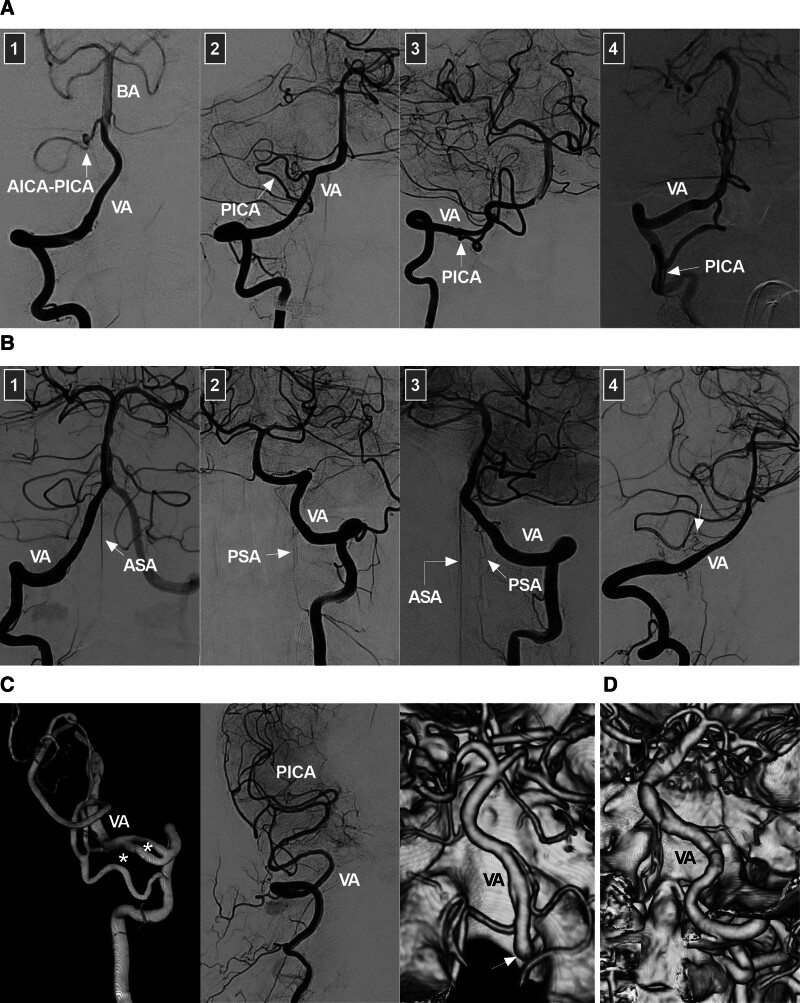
Intracranial VA anatomy and anomalies. (A) PICA anatomy: panel 1: DSA image showing the PICA and AICA sharing a common trunk (arrow); panel 2: DSA image showing the PICA (arrow) emerging from the distal VA; panel 3: DSA image showing the PICA (arrow) emerging from the intracranial proximal VA; panel 4: DSA image showing the PICA (arrow) emerging from the extracranial VA. (B) ASA and PSA anatomy: panel 1: DSA image showing the ASA (arrow) emerging from the VA; panel 2: DSA image showing the PSA (arrow) emerging from the VA; panel 3: DSA image showing the ASA and PSA (arrows) emerging from the VA; panel 4: DSA image showing a perforating artery (arrow) emerging from the VA. (C) VA anomaly: left panel: DSA image showing 2 fenestrations (asterisks) of the VA; middle panel: DSA image showing a VA terminating at the PICA; right panel: CTA image showing a VA emerging from the primitive hypoglossal artery (arrow). (D) CTA showing VA dolichoectasia, a form of vertebrobasilar dolichoectasia. AICA = anterior inferior cerebellar artery, ASA = anterior spinal artery, BA = basilar artery, CTA = computed tomography angiography, DSA = digital subtraction angiography, PICA = posterior inferior cerebellar artery, PSA = posterior spinal artery, VA = vertebral artery.
